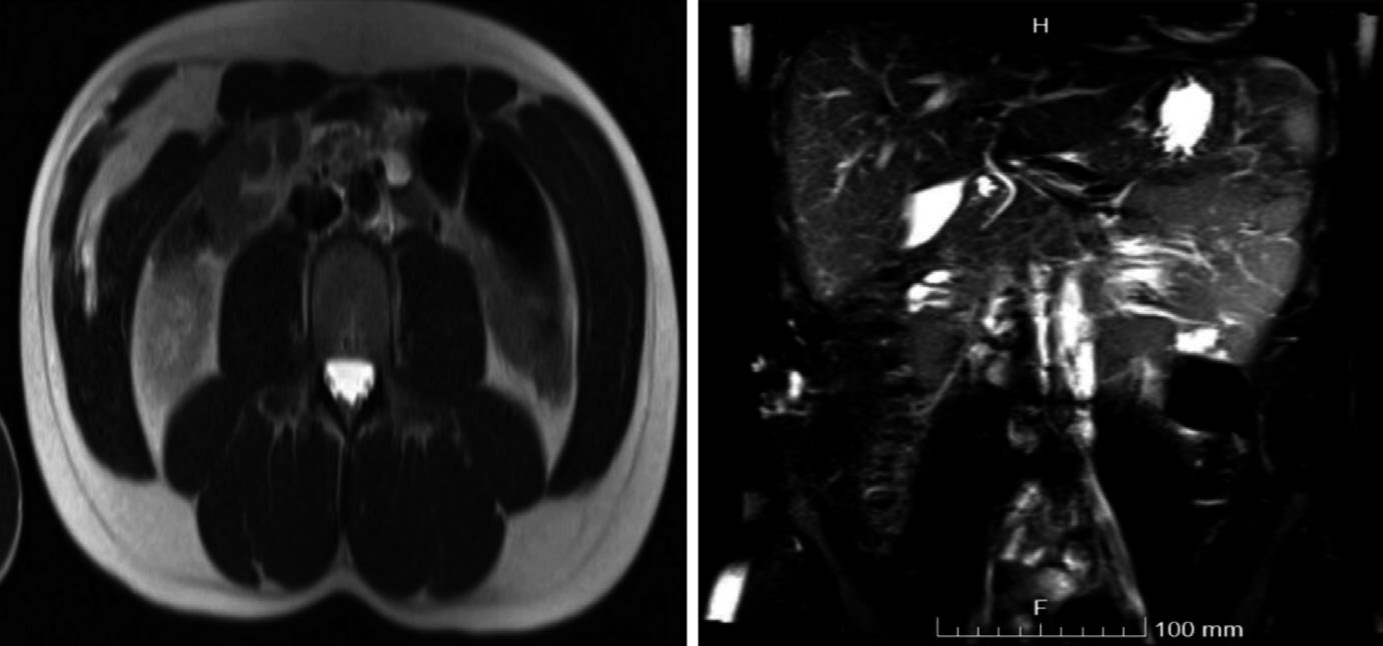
Magnetic resonance imaging of the abdomen with contrast (axial and coronal) showing a large intramuscular mass in the right anterior abdominal wall, extending from the lower edge of the costal margin to a few centimeters above the inguinal ligament (15 cm)
